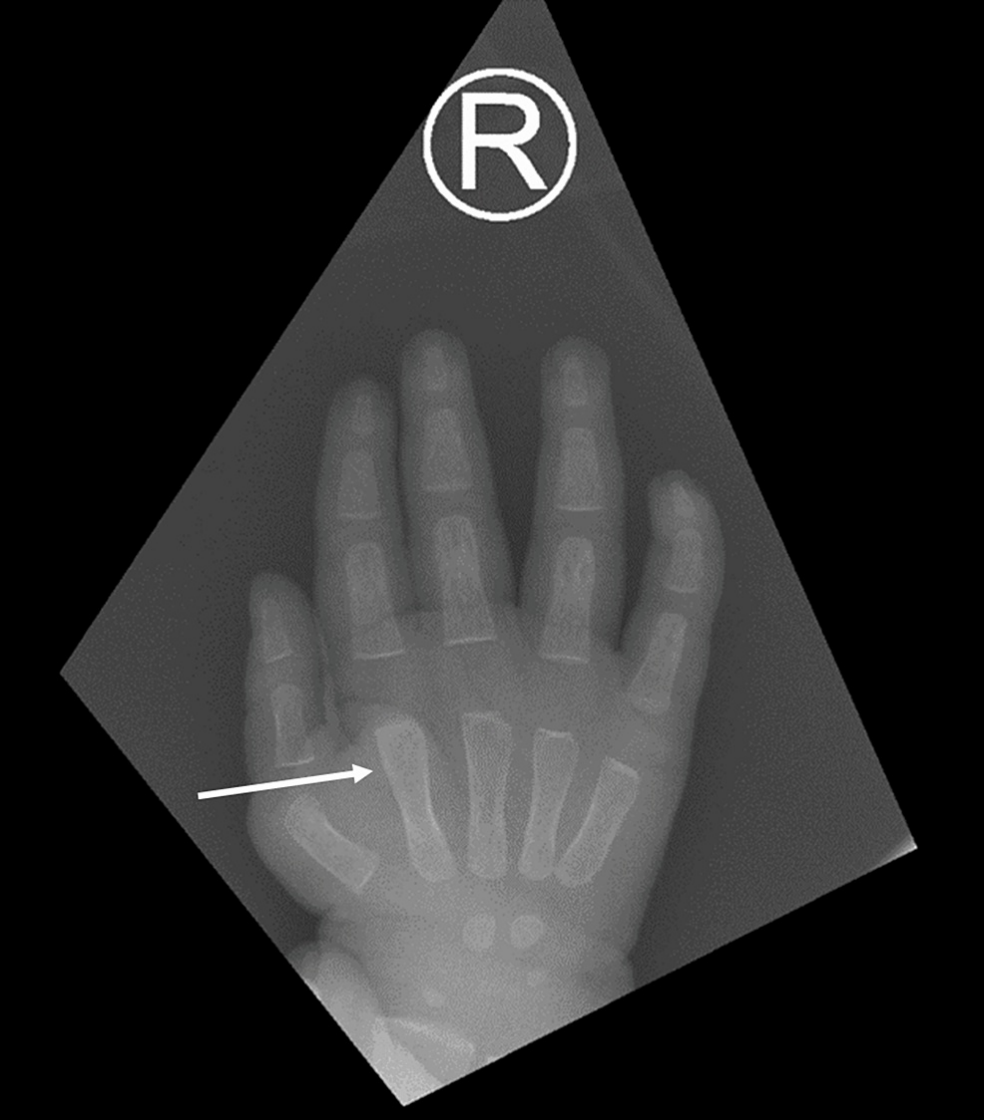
An X-ray of the right hand showing a mild widening of the short bones.
